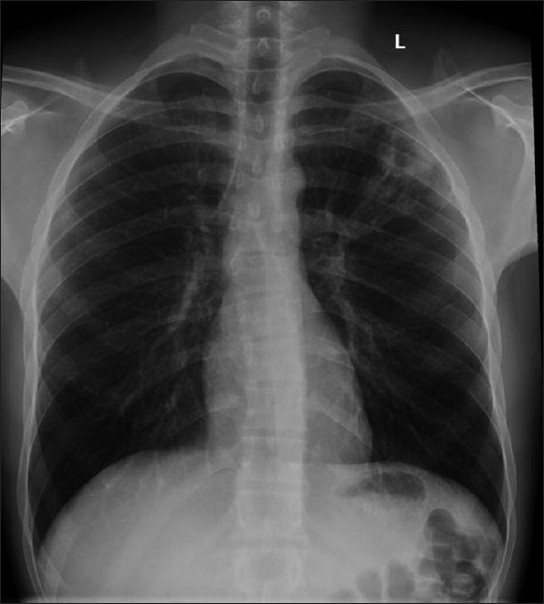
Cryptococcosis. Chest X-ray shows a less-frequently seen cavitation due to Cryptococcosis in an acquired immunodeficiency syndrome patient
